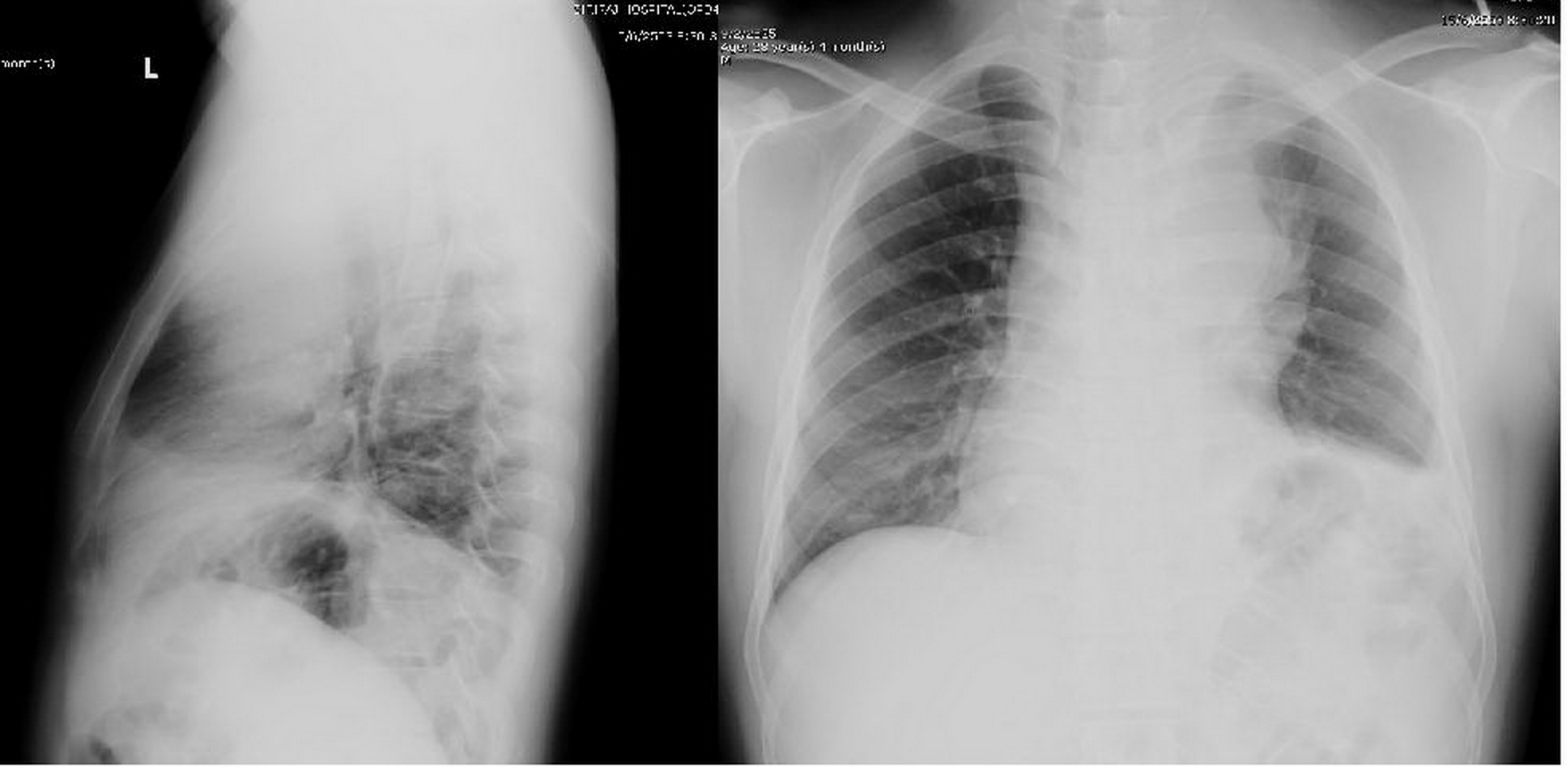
Chest X-ray revealed a large anterior mediastinal mass.
